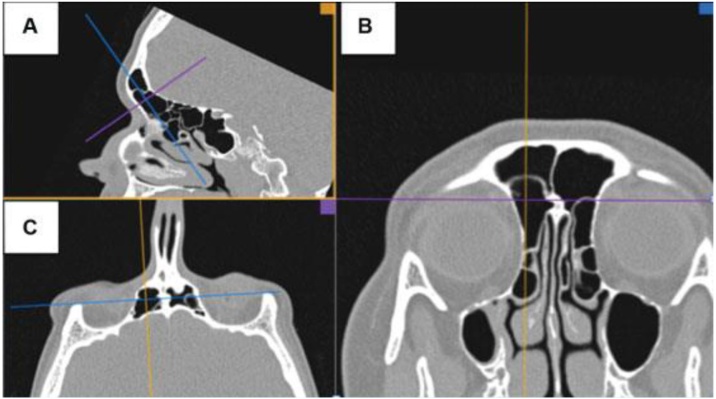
Multiplanar tomographic reconstruction. Multiplanar reconstruction of Computed Tomography (CT) scan using OsiriX DICOM viewer. (A) Parasagittal CT section, (B) Oblique section for true coronal plane, (C) Oblique section for true axial plane.
